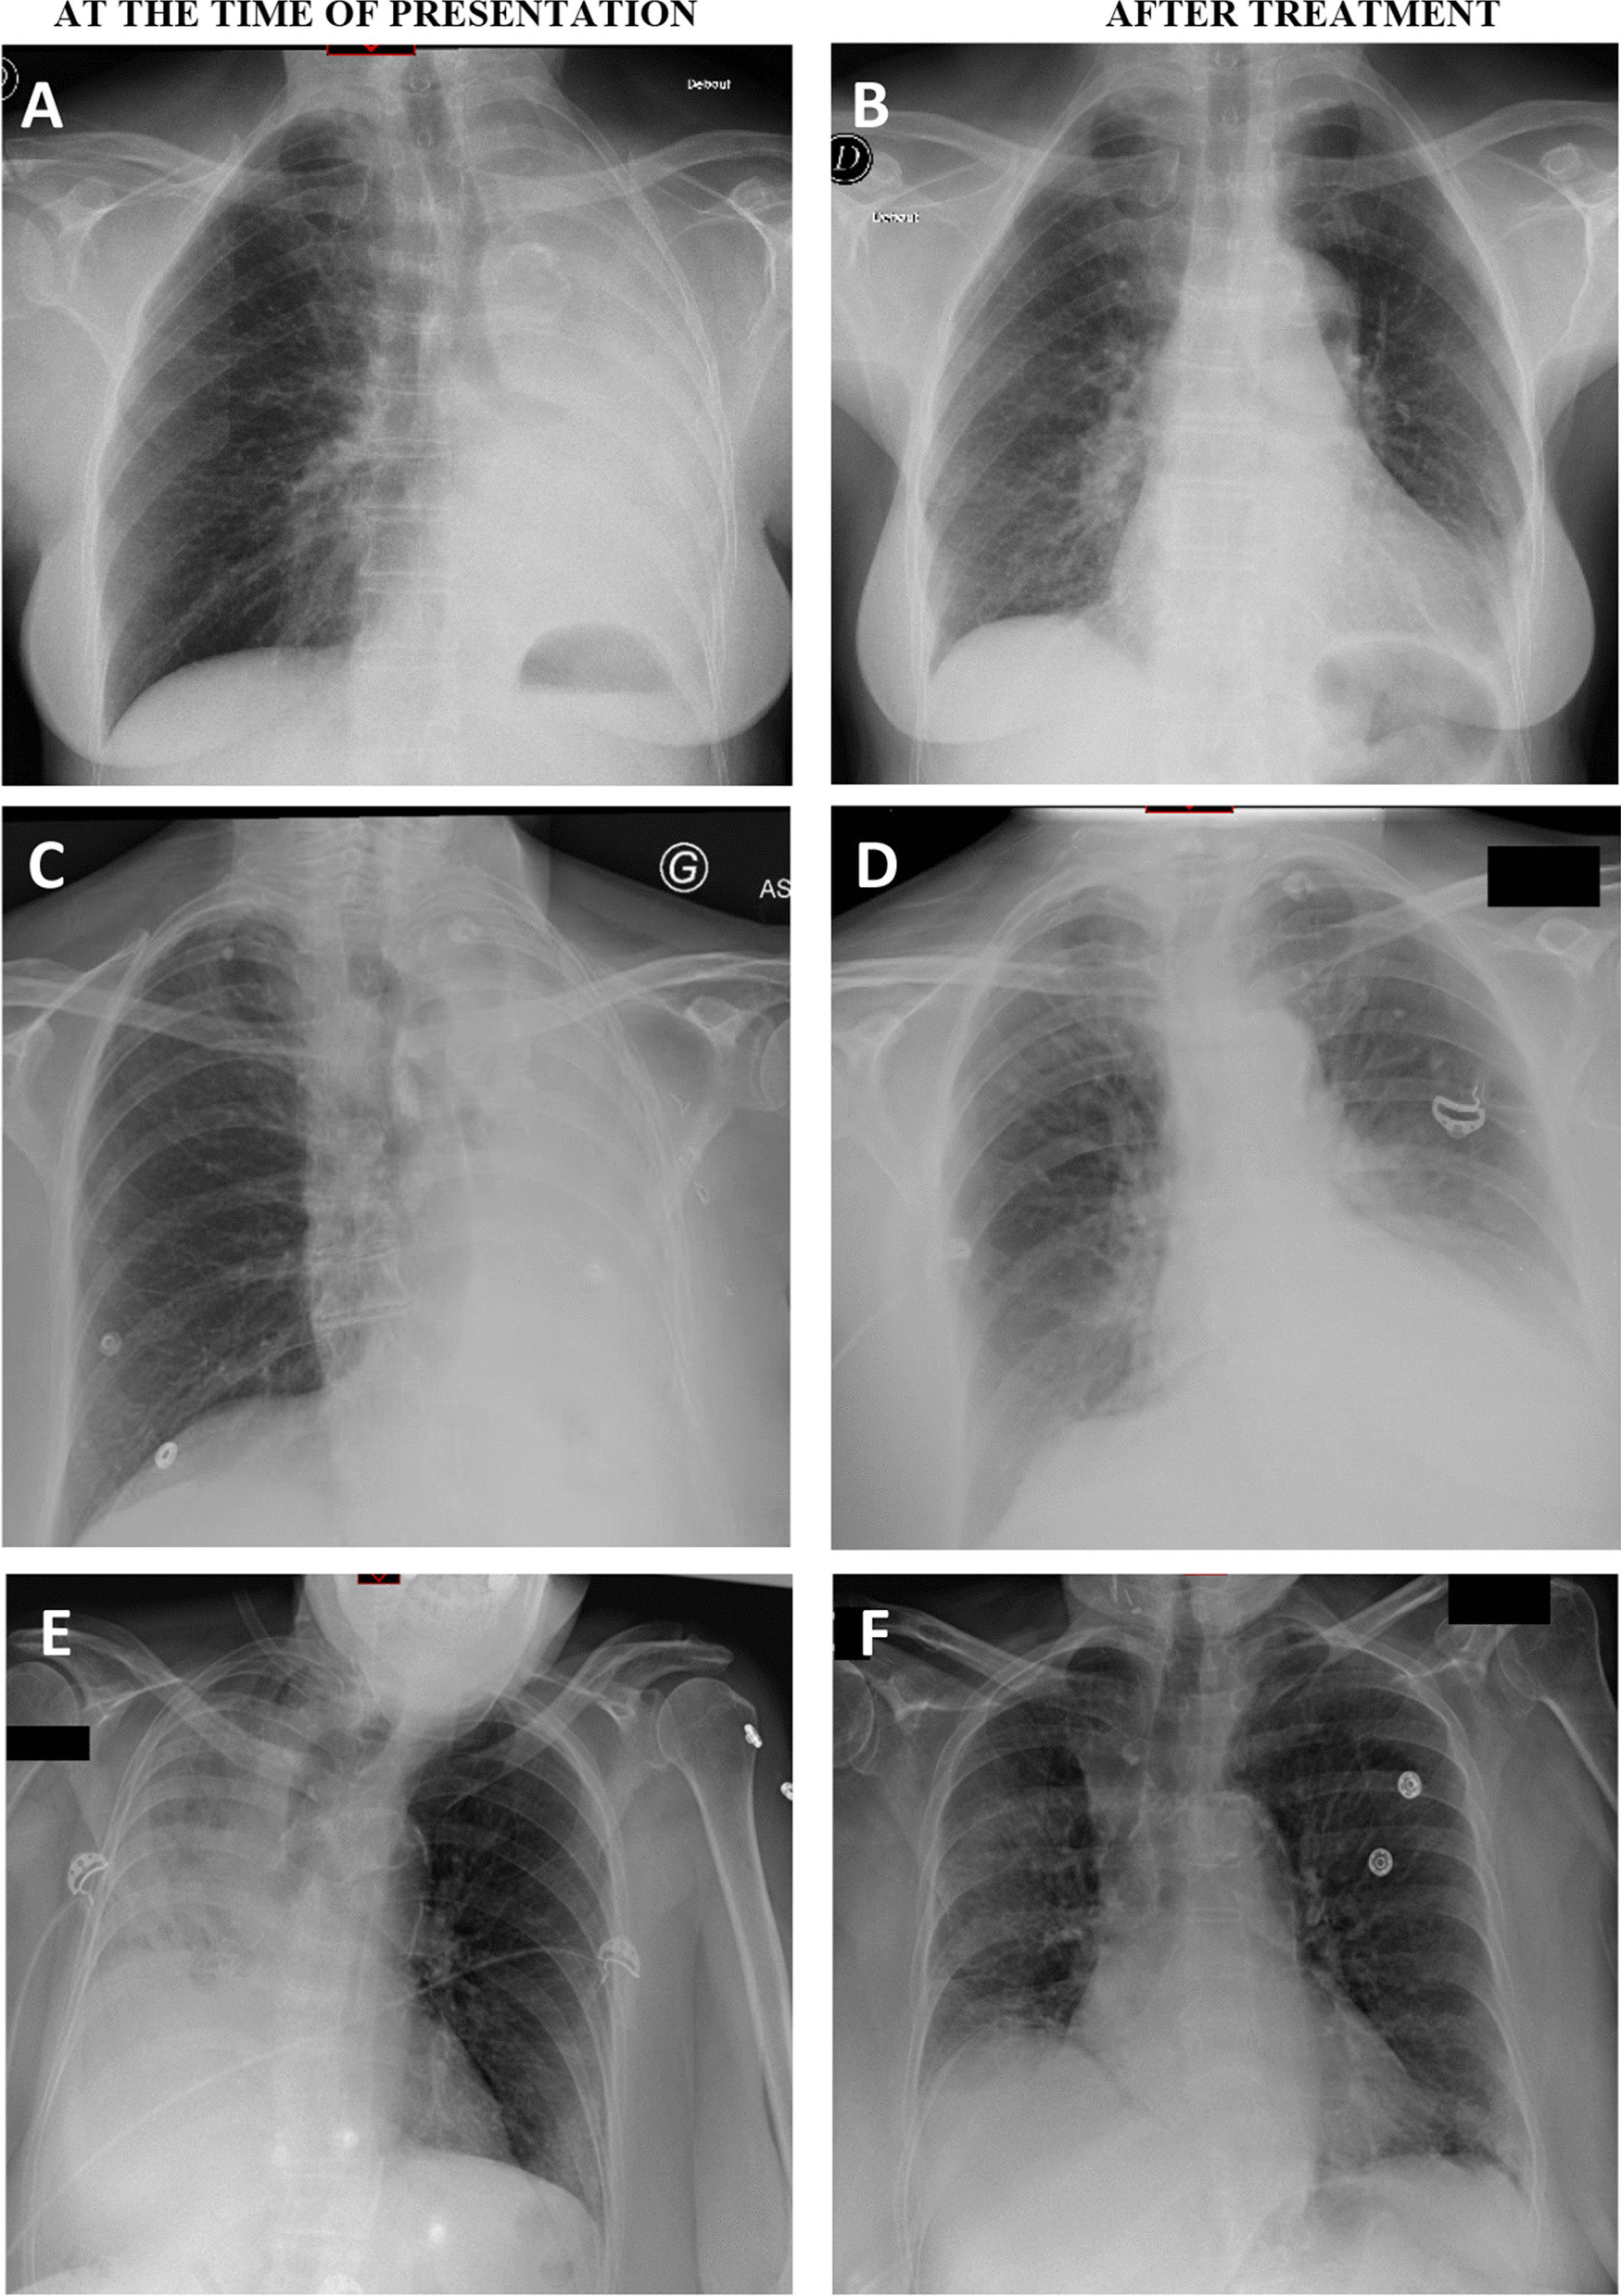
Chest X-ray of case #2 (A, B), #4 (C, D), and #5 (E, F) at the time of initial presentation, showing total lung atelectasis (A, C, E), and after treatment with flexible bronchoscopy and corticosteroids (B, D, F), showing a significant improvement
